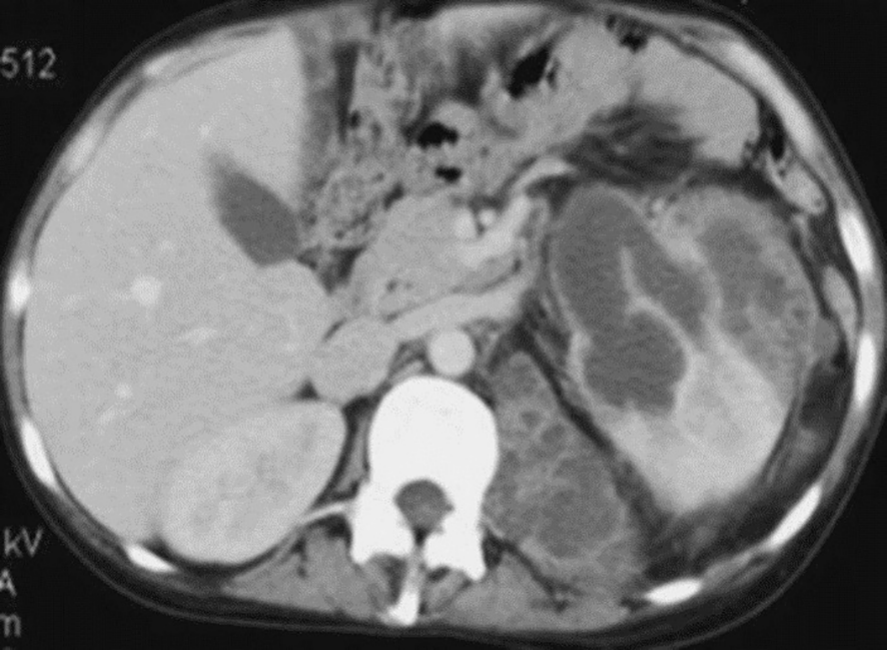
Contrast-enhanced abdominal CT scan showing a multilocular right lower-pole renal mass (white arrow). Scale bar = 1 cm.
